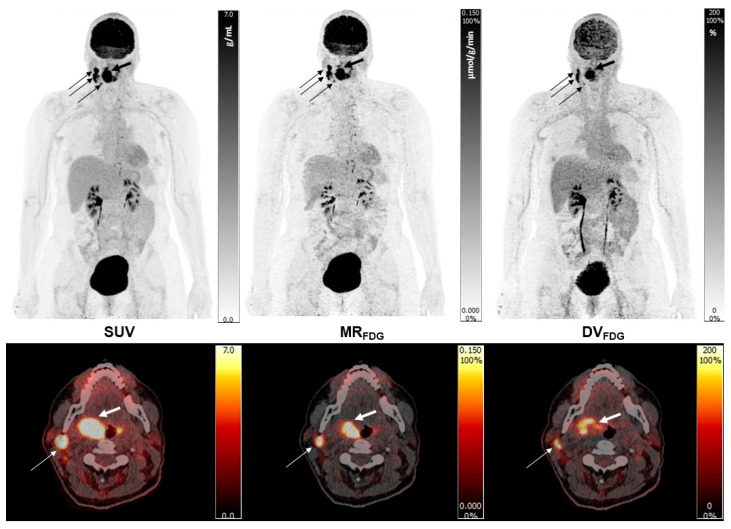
Example of SUV_bw_, MR_FDG_, and DV_FDG_ parametric images in a patient with right-sided oropharyngeal squamous cell carcinoma (SCC; thick arrows) and local lymph node metastases in neck levels II–IV (thin arrows). MR_FDG_ represents the irreversible uptake rate constant reflecting phosphorylated [^18^F]FDG-6-phosphate accumulation, while DV_FDG_ reflects the fractional volume of freely exchangeable, non-phosphorylated tracer and blood-pool activity. Together, these images demonstrate complementary kinetic information beyond static SUV_bw_ data.
